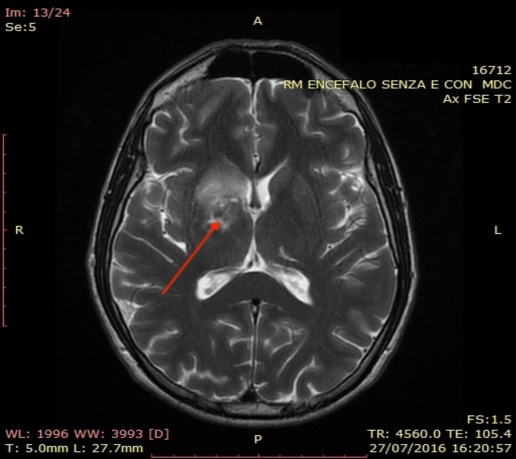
Brain MRI (T2‐weighted sequence). The arrows point to n hyperintensity area mainly involving thalamic and lenticular caudate with extension to internal and periventricular capsule. It is likely to be a subacute ischemic lesion. Day 2 after second episode of diving session.
